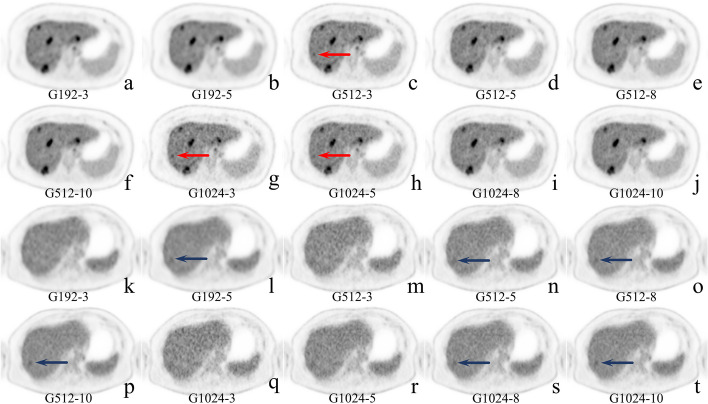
Typical cases with false-positive and false-negative lesions. A 35-year-old female was diagnosed with liver metastases from rectal cancer (a–j). G512-3 (c), G1024-3 (g), and G1024-5 (h) showed a positive lesion that was not present on images from longer acquisition times and MR images. It was identified as a false positive. A 58-year-old male was diagnosed with adenocarcinoma of the colon with multiple metastases to the liver (k–t). G192-5 (c), G512-5 (n), G512-8 (o), G512-10 (p), G1024-8 (s), and G1024-10(t) showed a positive lesion in the right lobe of the liver(pointed by blue arrows), which could not be seen in the rest of sub-figures. It was identified as a false negative in G192-3 (k), G512-3 (m), G1024-3 (q), and G1024-5 (r)
